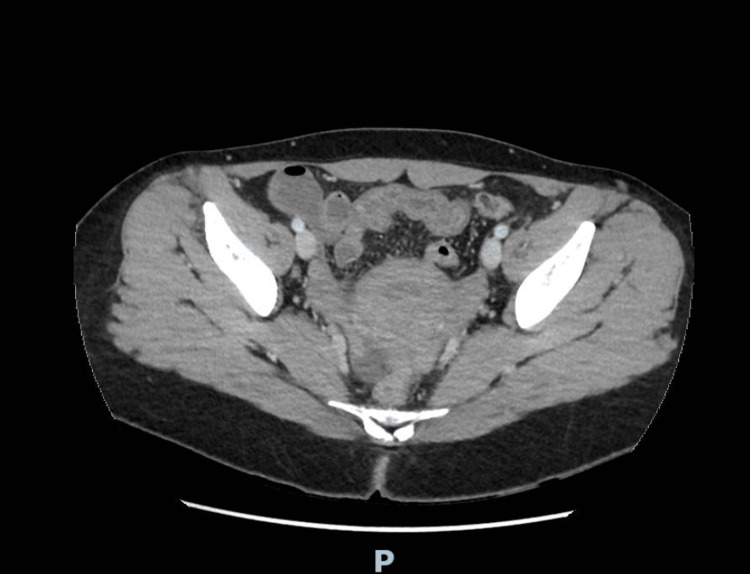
Axial contrast-enhanced CT image of the pelvis. No acute pelvic abnormality or free fluid is identified.
